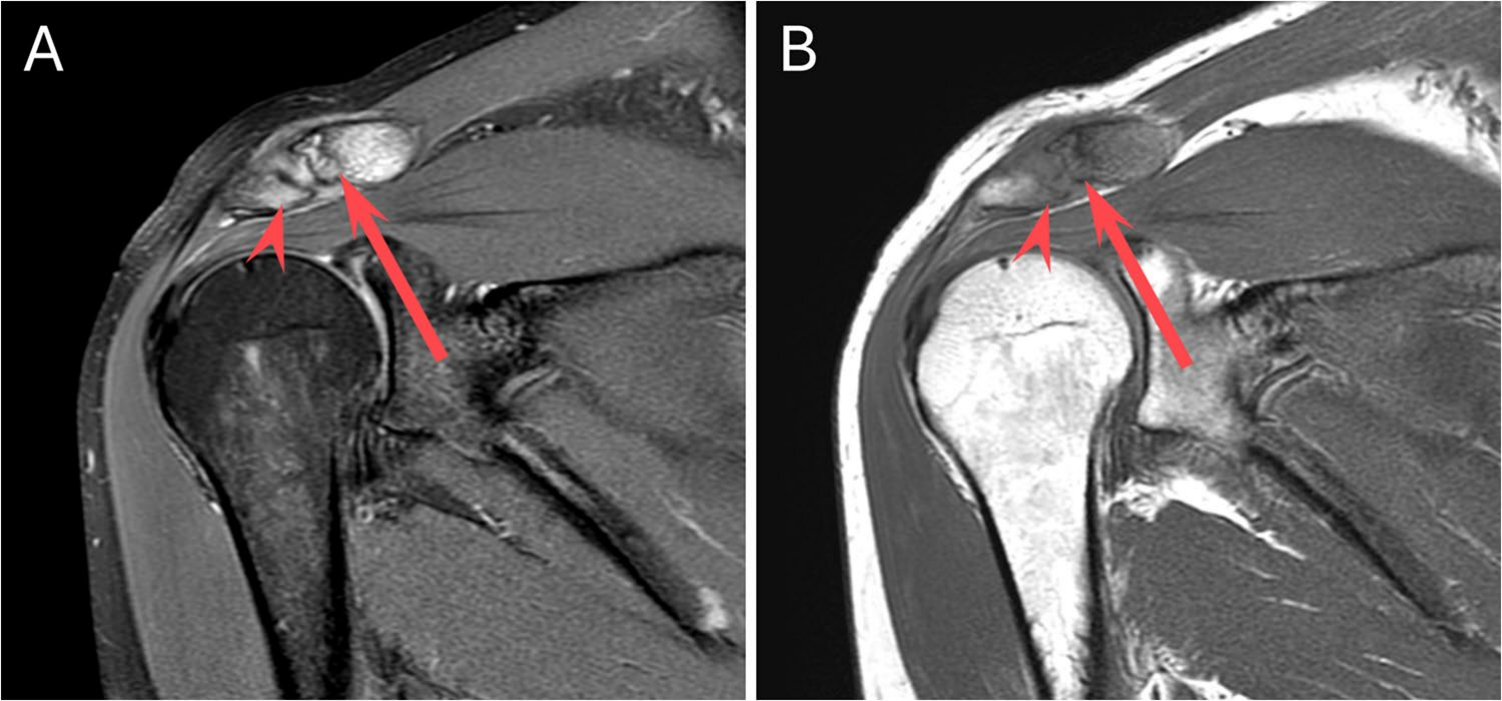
A 46-year-old delivery truck driver with acute-on-chronic right shoulder pain related to overuse. Coronal STIR (A) and coronal T1 (B) MR images demonstrate findings of osteolysis both in the distal clavicle (arrow, A and B) and in the anterior acromion (arrowhead, A and B)
